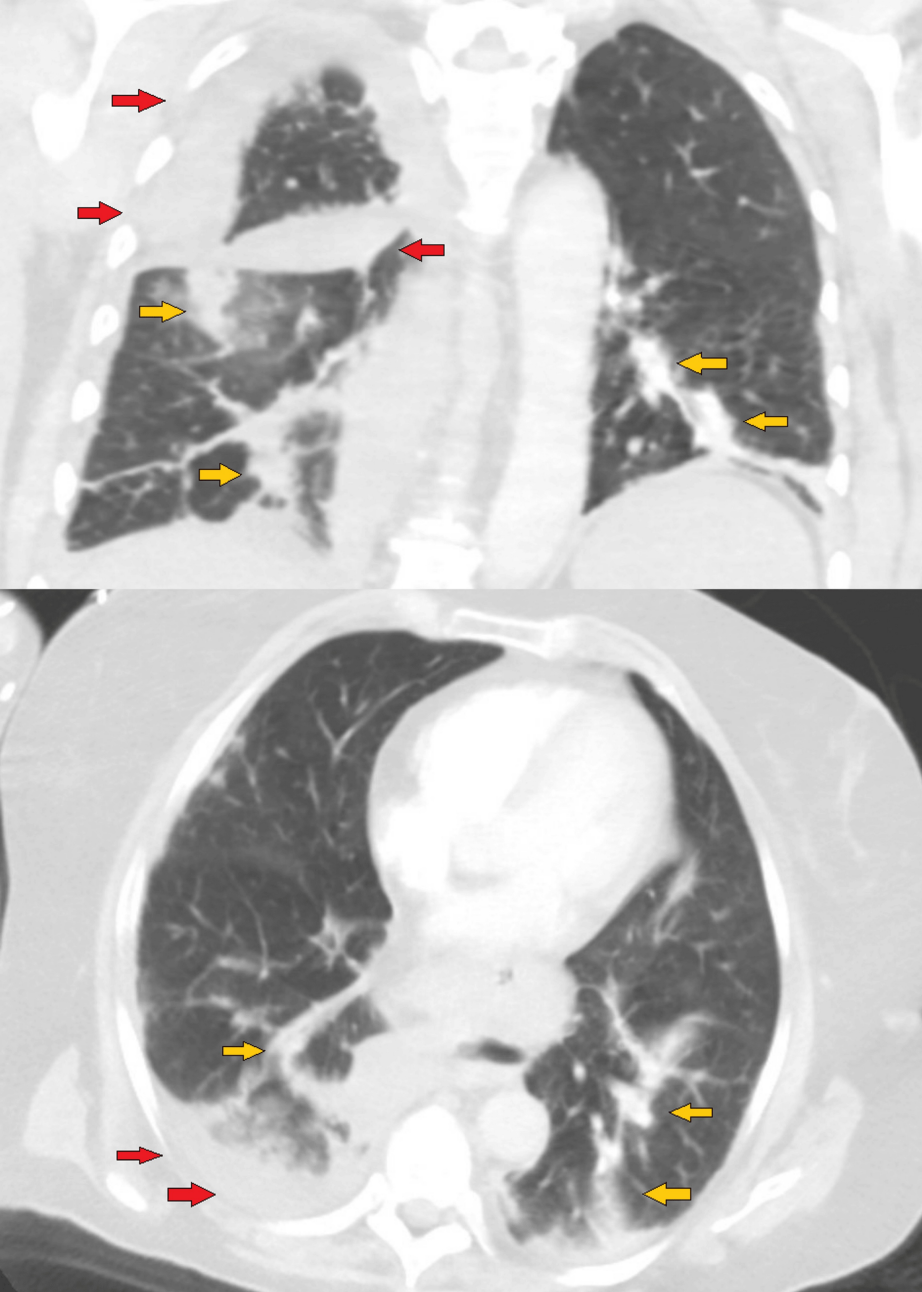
Pulmonary infarction & pleural effusions on computed tomography angiography (CTA) chest Emergency department CTA chest (lung windows) of coronal (top) and axial (bottom) cuts showing pulmonary infarct (yellow arrows) and pleural effusions (red arrows). Formal radiologist interpretation: "Extensive right pulmonary emboli. No CT evidence of right heart strain. Wedge-shaped consolidations in the right middle lobe and right lower lobe suspicious for developing infarct. Small right pleural effusion."
